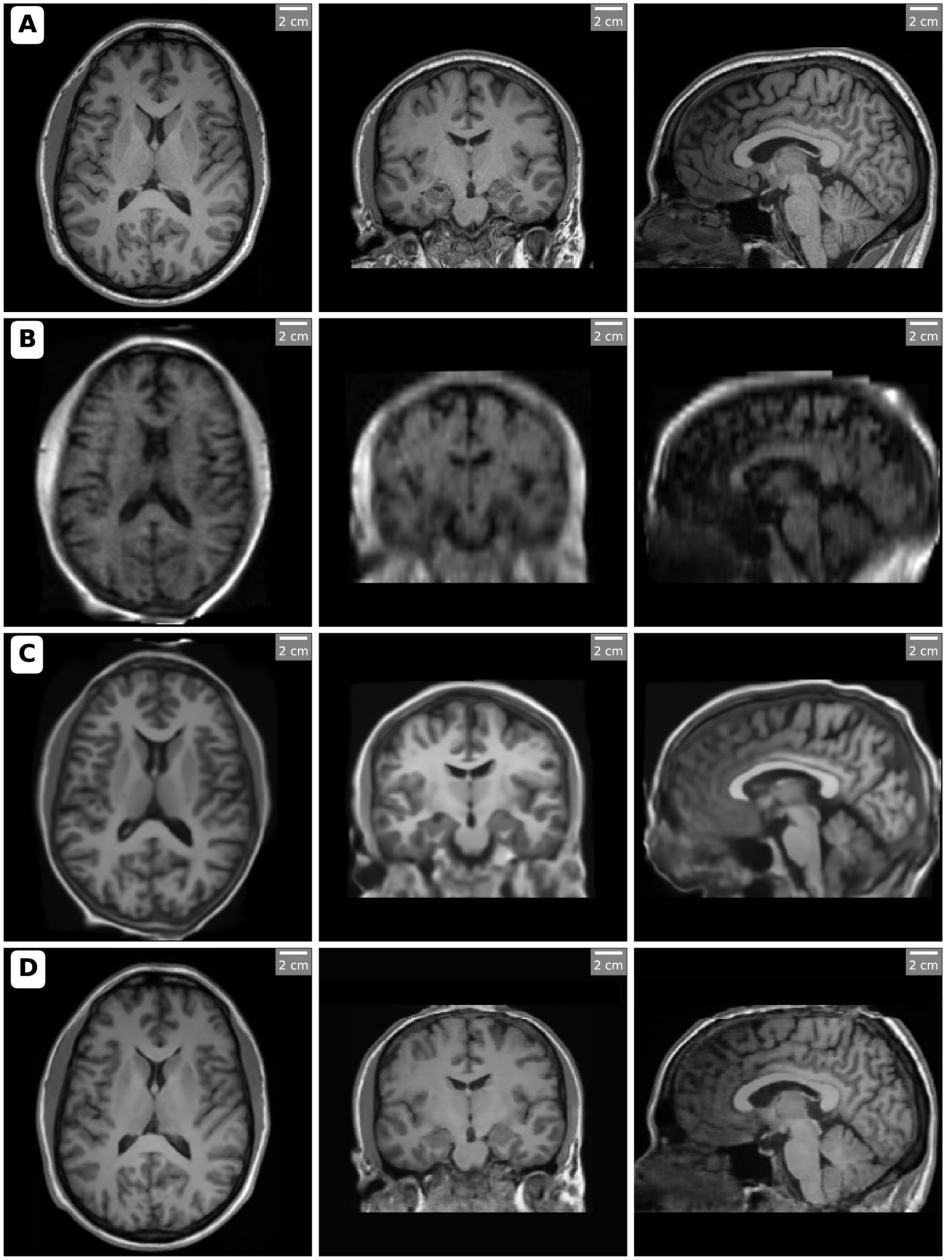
Orthogonal views (axial, coronal, and sagittal slices) of MRI scans acquired and processed with different methods. Each row corresponds to a specific imaging method: (A) High-field MRI (3T), (B) Ultra-low-field MRI (64mT), (C) SynthSR-enhanced MRI, and (D) LoHiResGAN-enhanced MRI. The slices highlight the differences in image quality and anatomical clarity, with SynthSR and LoHiResGAN demonstrating significant enhancements in resolution and contrast for ultra-low-field MRI scans. Notably, in the SynthSR-enhanced images, artificially increased gray matter contrast is observed in certain regions, such as the frontal lobes, likely due to the model's integration of T1 and T2 inputs and its aggressive contrast enhancement strategy.
